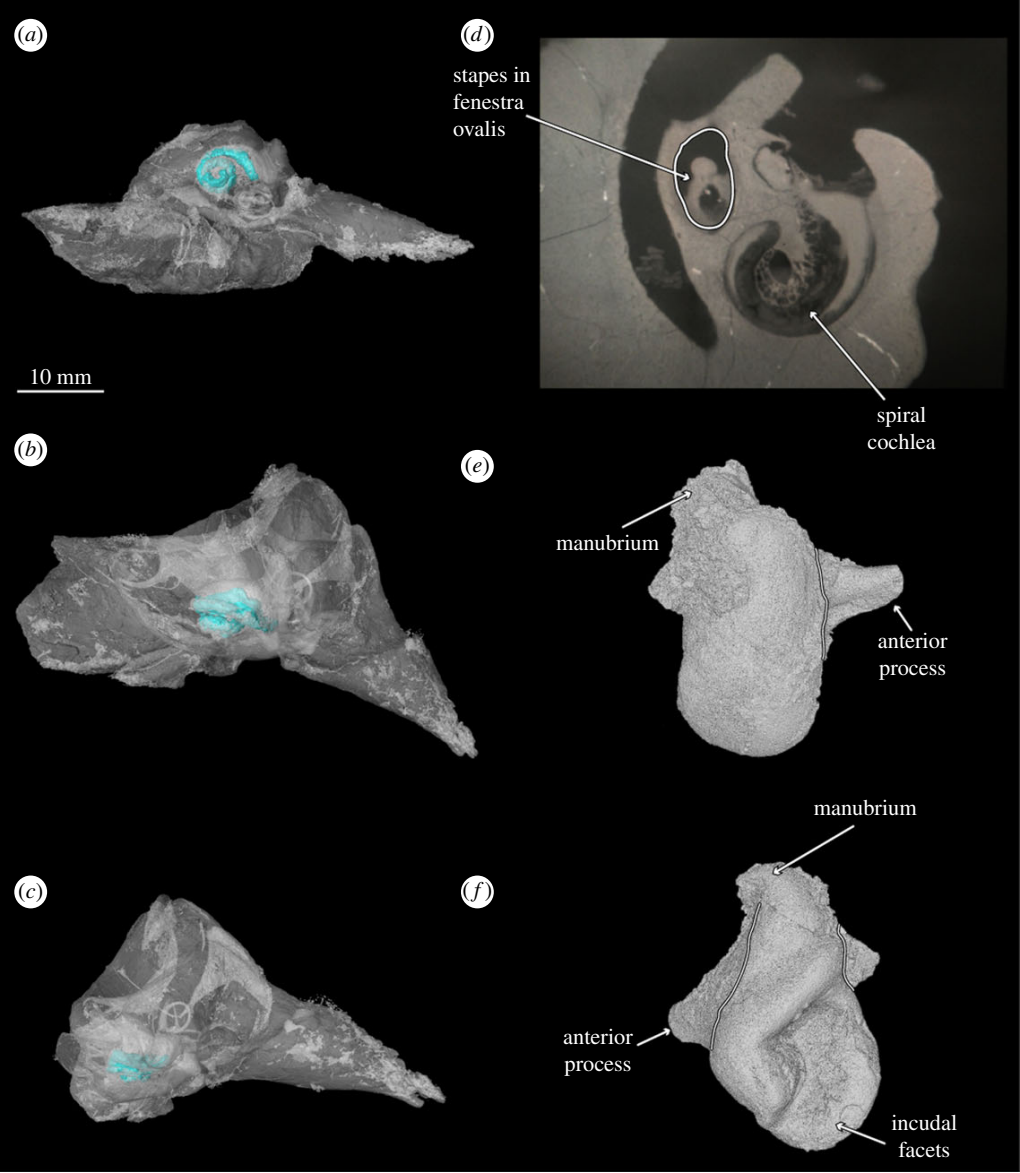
Three-dimensional models of holotype right periotic of *B. osedax* (USNM 539939) in ventral (*a*), medial (*b*) and anterior (*c*) views. The periotic is partially transparent to showcase the spiral cochlea (blue) *in situ* within the pars cochlearis. (*d*) Cross-sectional CT scan of the pars cochlearis revealing the spiral cochlea and the stapes *in situ* in the fenestra ovalis. (*e,f*) Three-dimensional models of the holotype right incus of *B. osedax* (USNM 539939).
